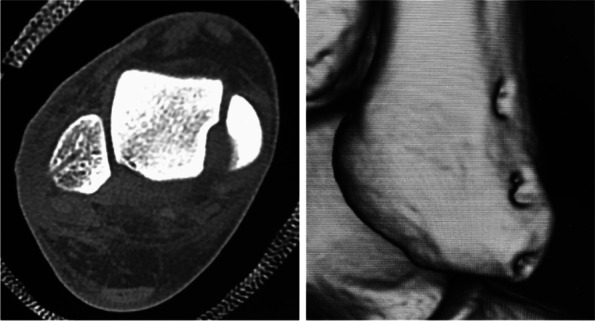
Postoperative CT image. Postoperative CT image showing the position and direction of the anchor holes
